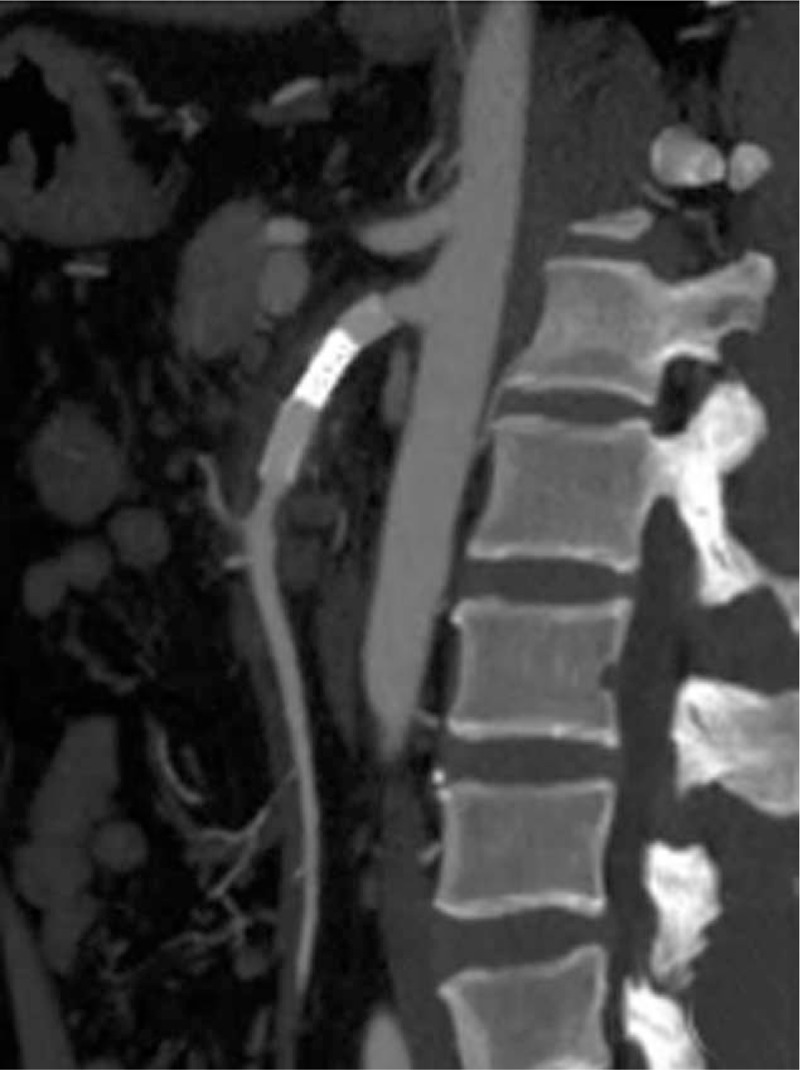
Computed tomography angiogram showing patency of the stent (arrow) 6 months postoperatively.
